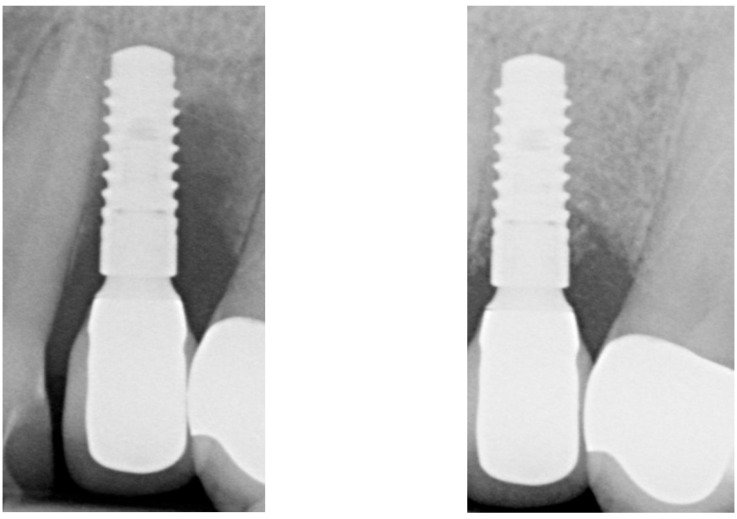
Baseline (T_Pre_, (left)) and postoperative (T_12_, (right)) radiographical situation, Case 1.
